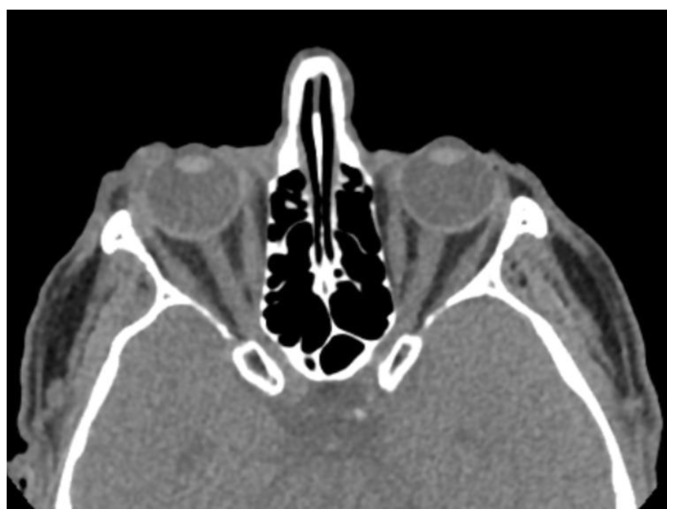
Patient with penetrating globe injury in the right eye. The patient has no CT findings and is classified in ocular trauma score category III.
